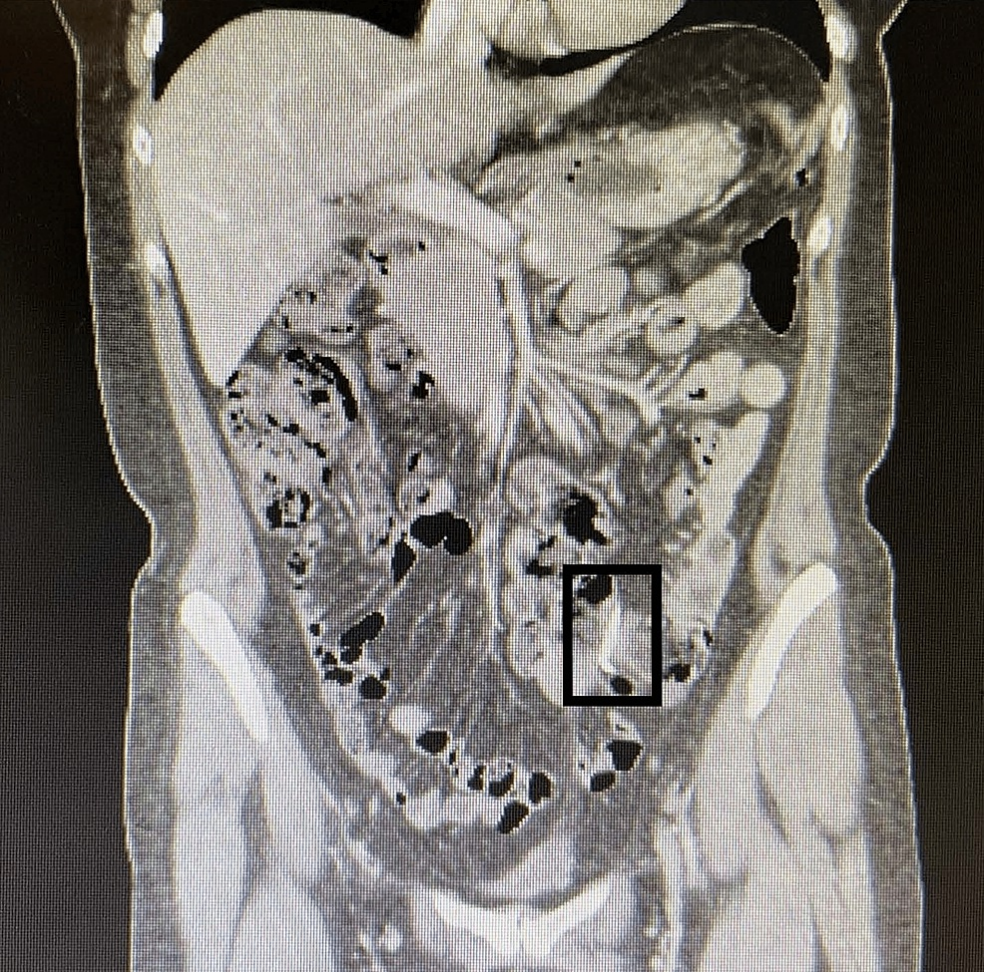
Computed tomography scan of the abdomen and pelvis without intravenous contrast demonstrating an extrauterine intrauterine device in the patient's left lower quadrant identified by a black rectangle
